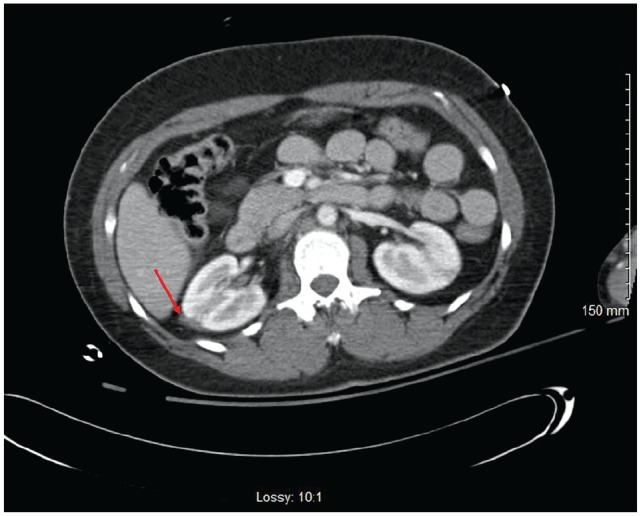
Computed tomography of abdomen with contrast demonstrating small amount of fluid in the right Gerota’s fascia (red arrow) with a subtle fracture of the upper pole of the right kidney.
